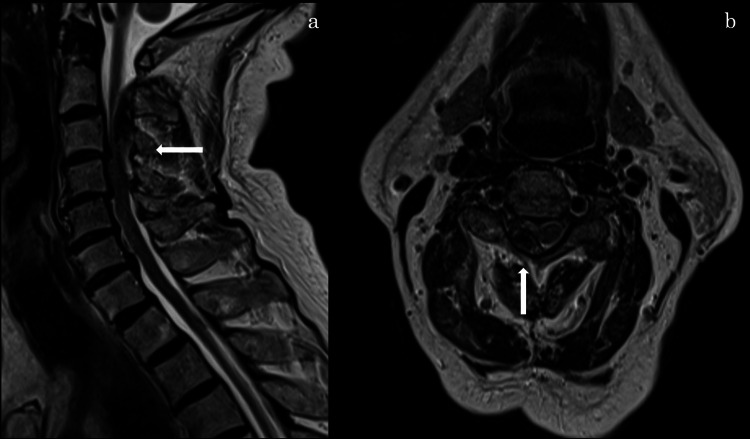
MRI T2-weighted image (a) T2-weighted sagittal image showing a hematoma located dorsally at the C2–C4 vertebral levels. (b) T2-weighted axial image showing an extradural hematoma compressing and displacing the spinal cord at the C2–C4 levels.
